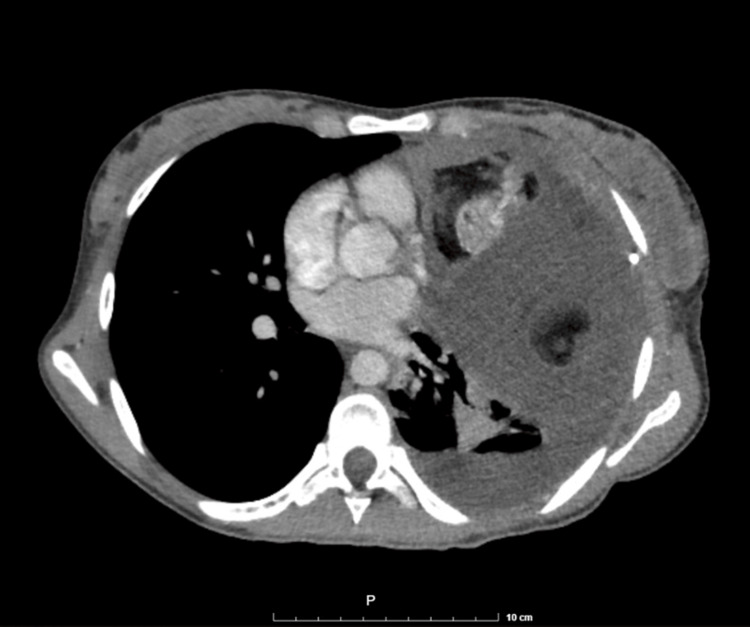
Preoperative chest computed tomography, axial view demonstrating cystic and calcified components.
